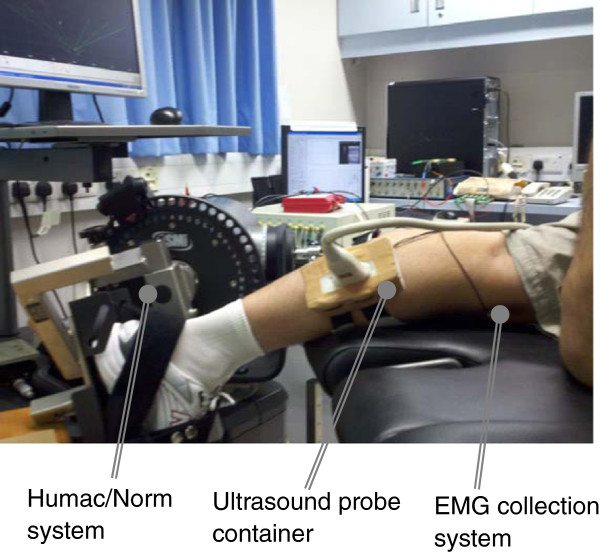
Experimental setup including EMG and ultrasound image data collection modules.
